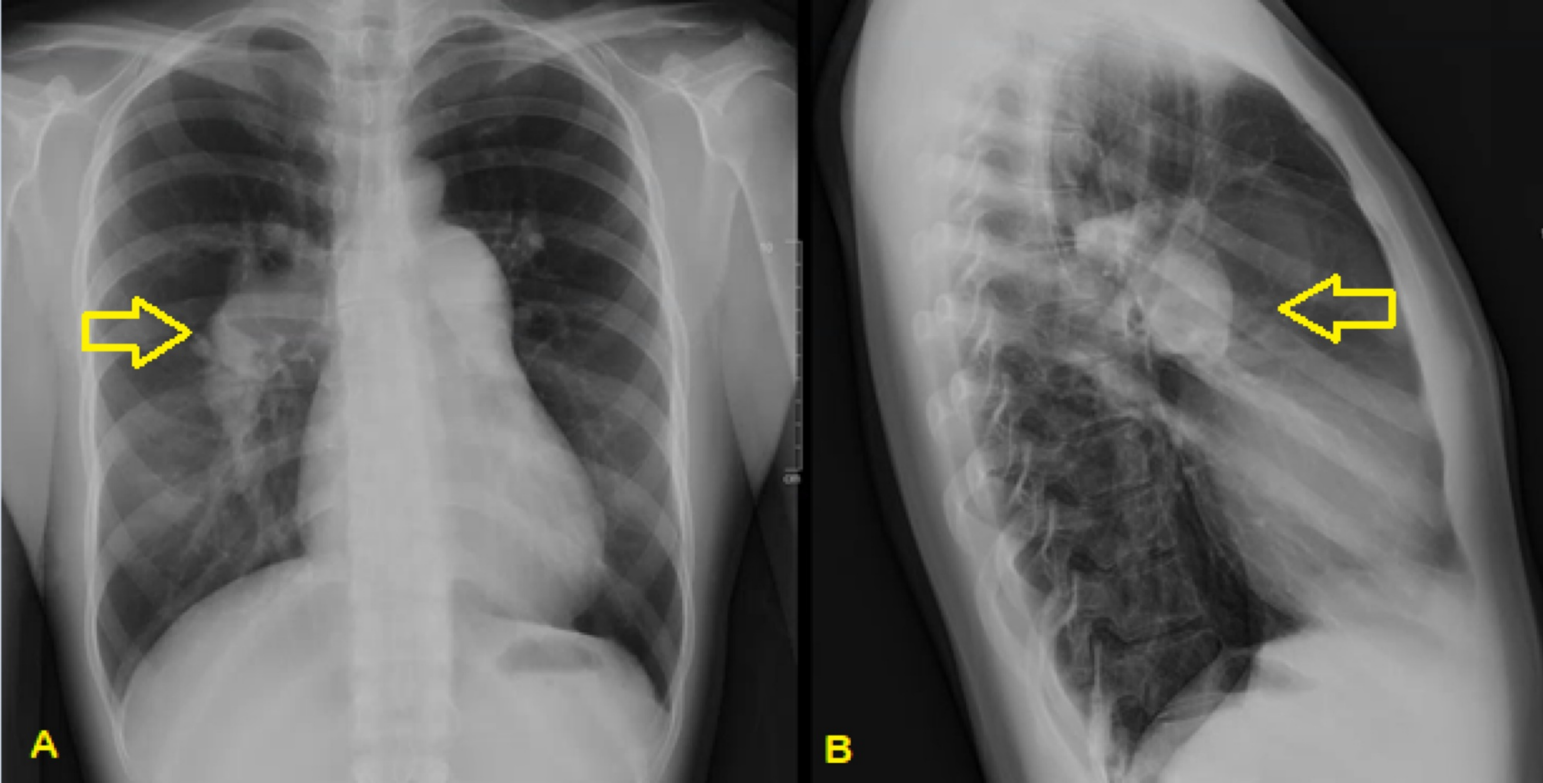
Anterior-posterior (A) and lateral (B) chest x-rays showing dilated pulmonary vasculature
